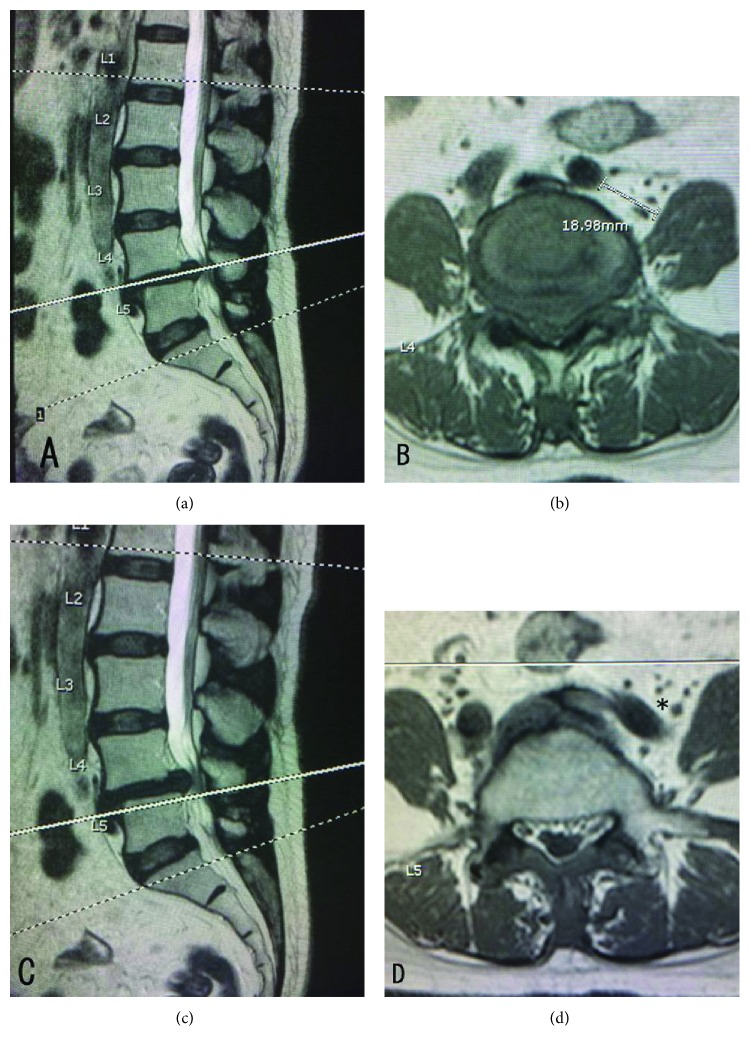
Preoperative MRI of lumbosacral spine T1W image showed prepsoas corridor at the level of intervertebral disc space L4-L5 (a and b) and at the level of upper vertebral body of L5 (c and d). Rising of psoas muscle was shown in (d). Left common iliac artery almost obliterates prepsoas space at level of upper vertebral body of L5 (black asterisk).
